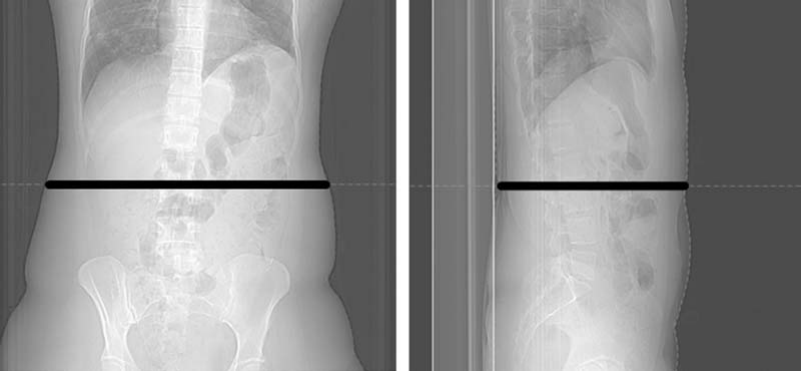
Localizer images for an abdominal scan; the black lines show the diameter of the patient in the central slice position; left: lateral direction (}{}$\mathrm{LAT}$); right: anterior–posterior direction (}{}$\mathrm{AP}$); the }{}$\mathrm{SSDE}$ values output by the CT scanner used in this study are calculated using this center-slice method.
